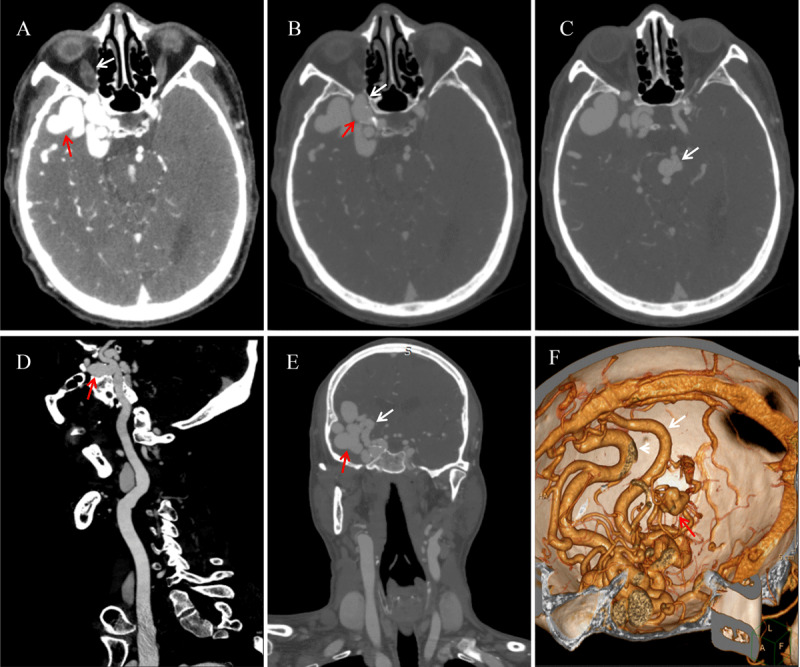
CTA presentation of intracranial arteriovenous malformation combined with multiple aneurysms.
